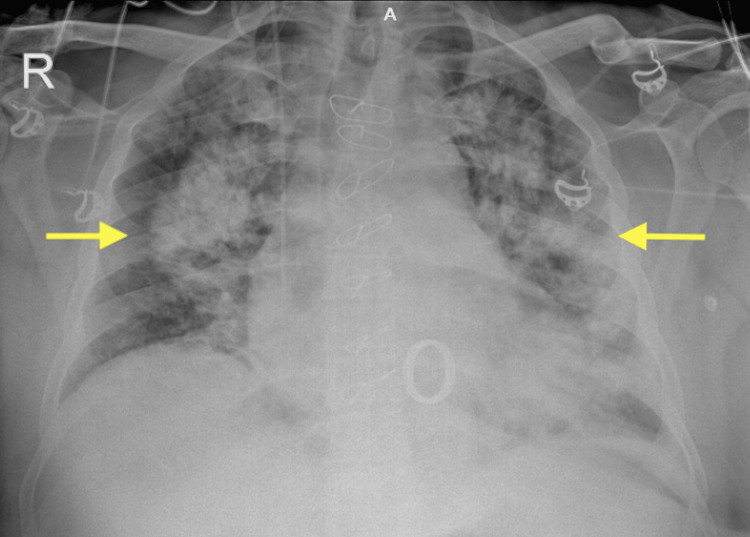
Multilobular bilateral pulmonary infiltration on chest X-ray
